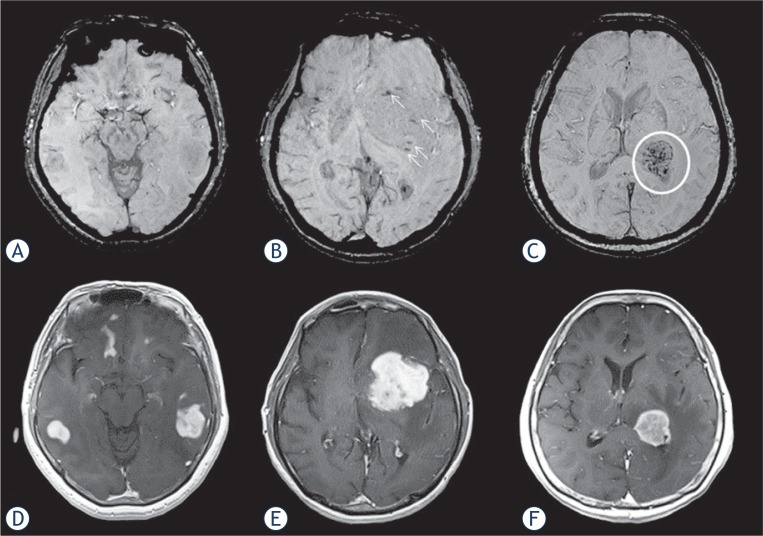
Intratumoral susceptibility signals in patients with primary central nervous system lymphoma: (A) Grade 1: multifocal tumors in bilateral temporal lobes show no intratumoral susceptibility signal on susceptibility-weighted imaging (SWI). (B) Grade 2: tumor in the left basal ganglia shows punctate low-intensity signals (arrows) on SWI. (C) Grade 3: tumor in the left thalamus shows multiple linear or nodular low-intensity signals (circle) on SWI. (D–F) Contrast-enhanced T1-weighted imaging shows primary central nervous system lymphomas with intense enhancement.
